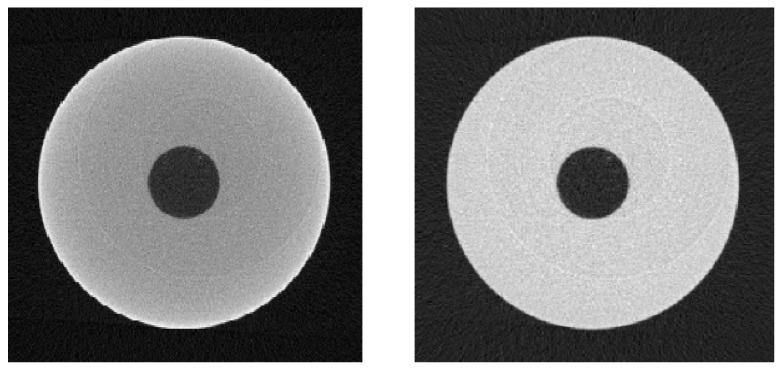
Transverse plane CT images of the aluminium workpiece. Left: uncorrected. Right: beam hardening corrected.
